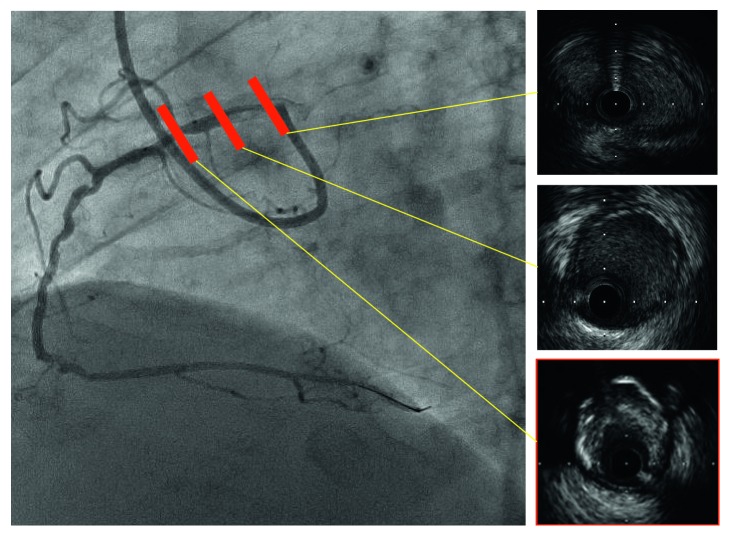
Intravascular ultrasound revealing diffuse eccentric calcified plaque, and the intramural course of the proximal ectopic artery was elliptical with some lateral compression.
